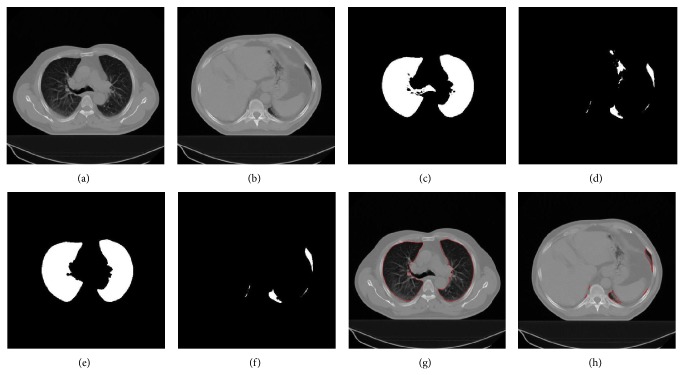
Segmentation of the lungs: (a, b) original CT slices, (c, d) rough segmentation of the lung fields of (a, b) in white, (e) lungs in white after eliminating the bronchi from (c), (f) lungs in white after removing intestine from (d), and (g, h) lung contours in red superimposed on the original slices.
